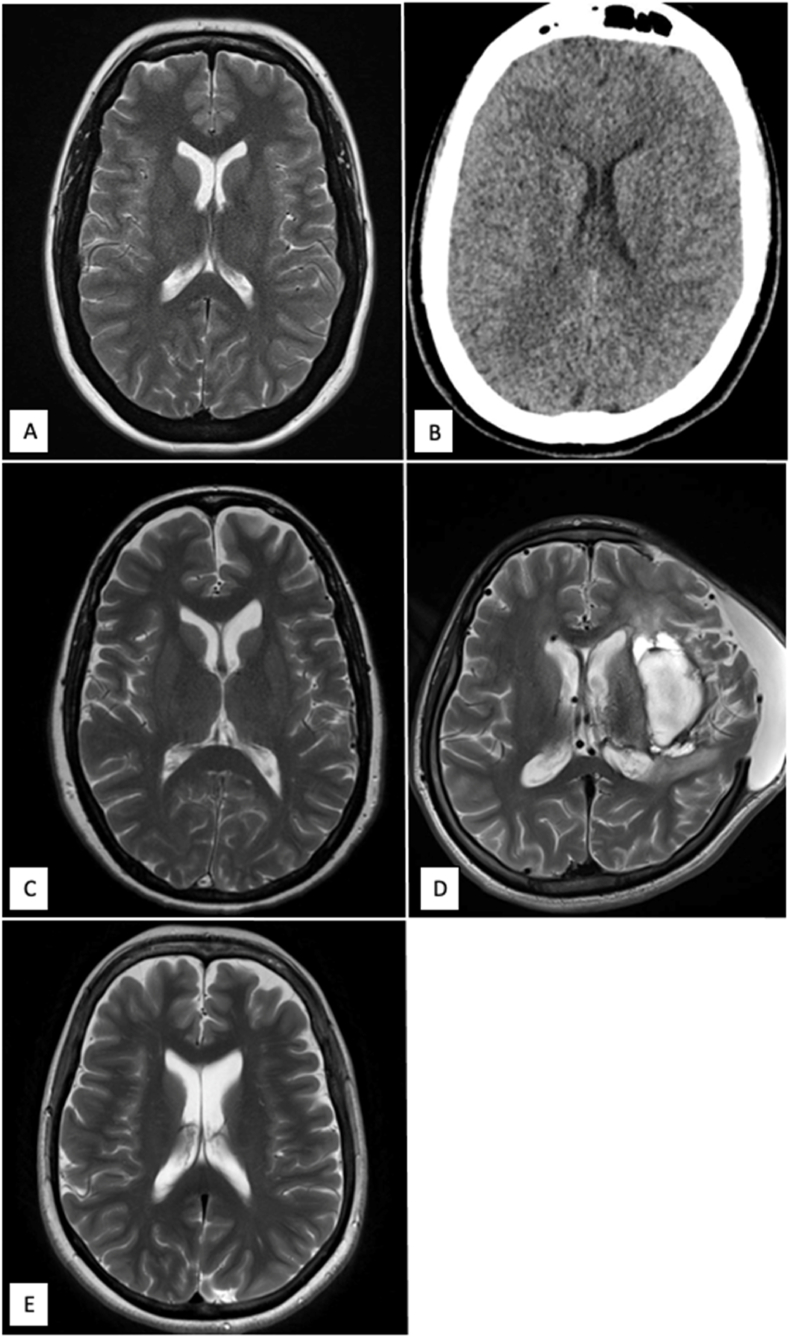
Head imaging of all patients (A to E), axial MRI or CT head. Mostly no gross abnormality, except patient D who had a previous bleed from an arteriovenous malformation with a decompressive craniectomy.
